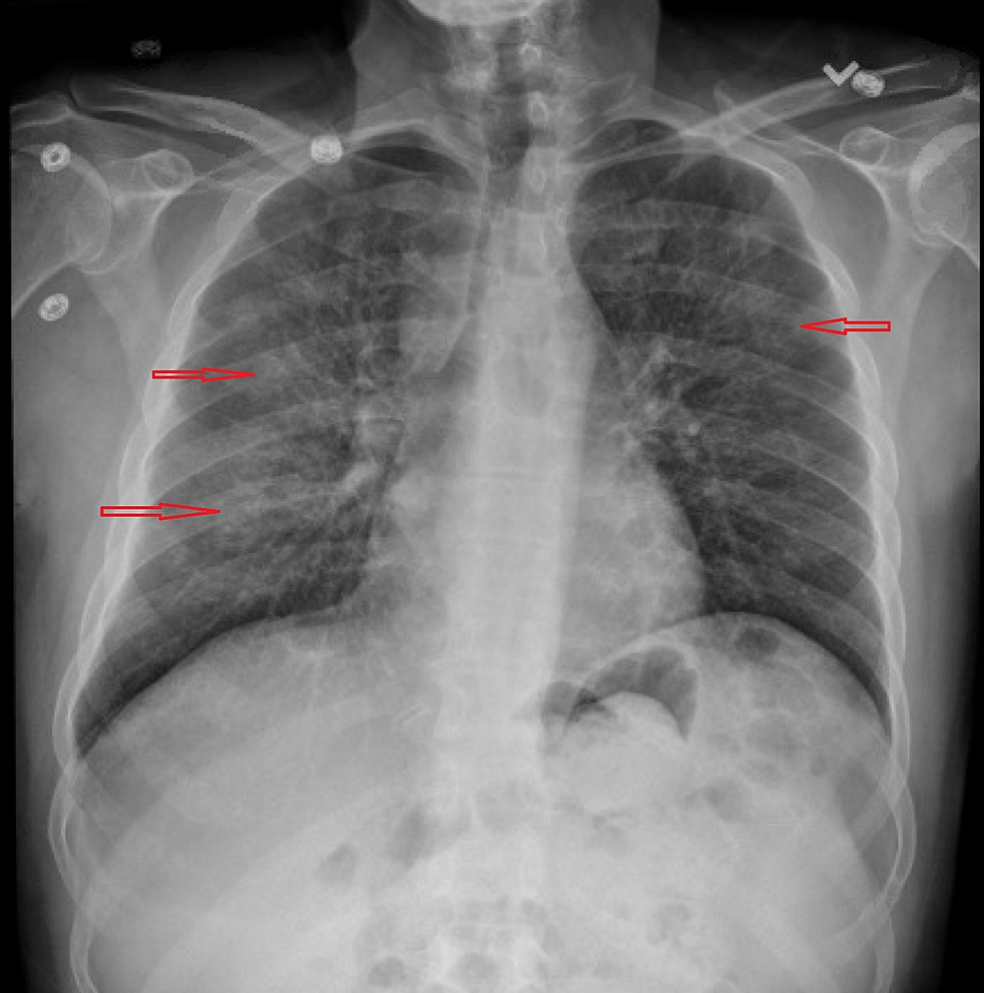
Chest X-ray demonstrating mild diffuse hazy opacities (red arrows) throughout both lung fields
